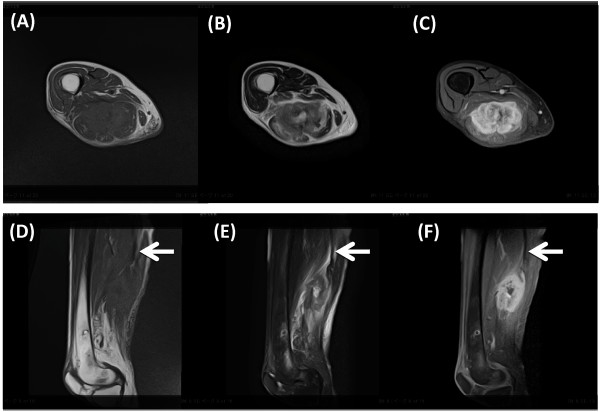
MRI of the thigh. Upper sections represent sagittal views, lower sections axial views. (A) (D) T1-weighted, (B) (E) T2-weighted, and (C) (F) enhanced T1-weighted images. MRI showed the mass arising from sciatic nerve (arrow) with low signal intensity on T1-weighted images, and heterogeneous high signal intensity on T2-weighted images and homogeneous high signal intensity on enhanced T1-weighted images.
